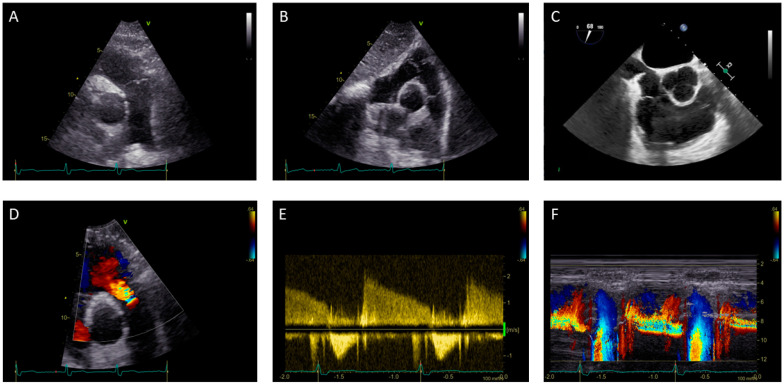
(A–C) Assessment with transthoracic and transesophageal echocardiography of the pulmonary valve in a 50-year-old female patient: (A) Parasternal short-axis view with focus on pulmonary valve and pulmonary artery; (B) Subcostal view; (C) Mid-esophageal right ventricle inflow-outflow view. (D–F) Transthoracic echocardiography assessment of pulmonary regurgitation in a 60-year-old male patient: (D) Detection of pulmonary regurgitation by color flow Doppler imaging; (E) Continuous wave Doppler of pulmonary regurgitation flow; (F) Color M-mode demonstrates holodiastolic regurgitation jet.
